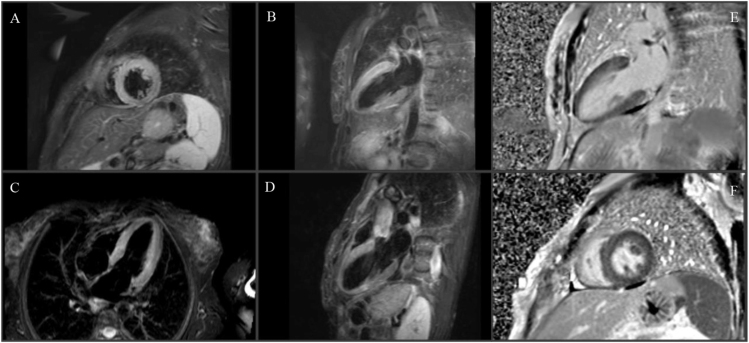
(A, B, C, D) T2-weighted images (STIR) showing hypersignal more evident in the basal and middle segments of the left ventricular anterior and anterolateral walls. (E and F) High-resolution late gadolinium enhancement (LGE) images showing no areas suggestive of the presence of myocardial necrosis or fibrosis.
